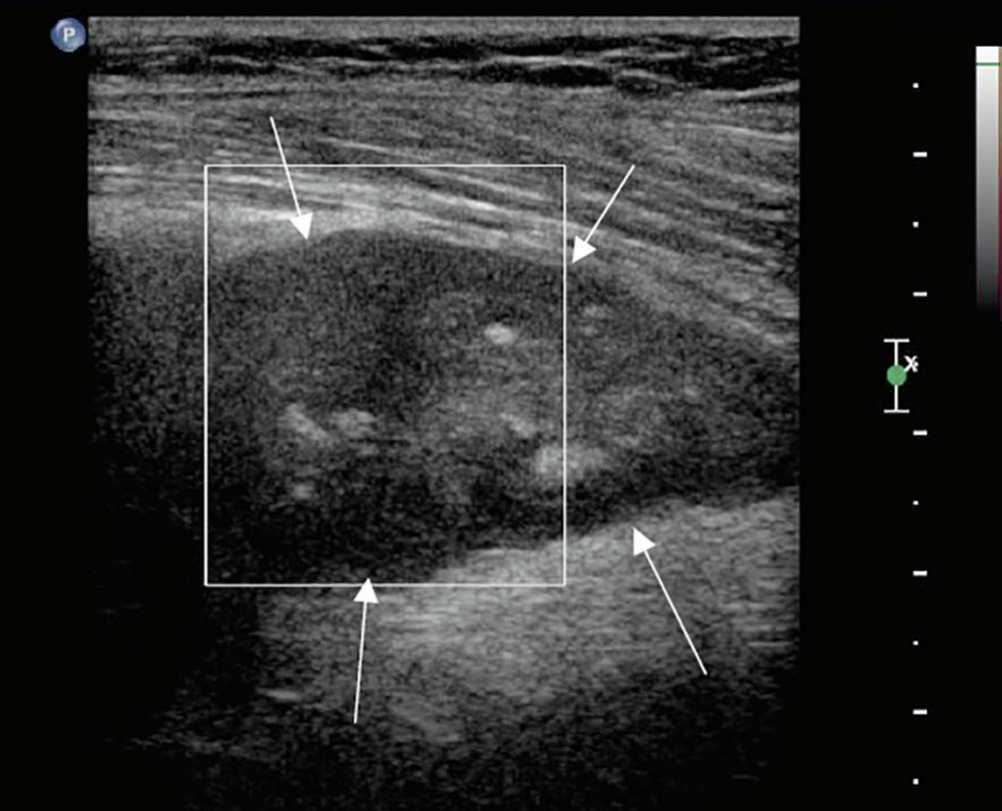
Local ultrasound shows a well-defined mass with a heterogeneous aspect with contact to the sciatic nerve (white arrows show the limit of the tumor).
